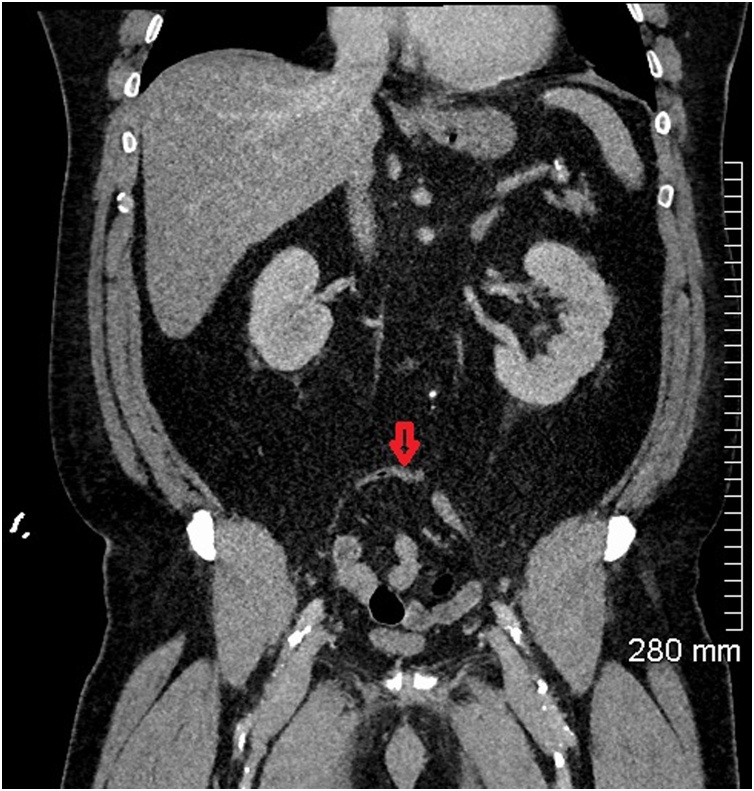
Coronal CT abdomen showing a normal appendix on admission (red arrow).
